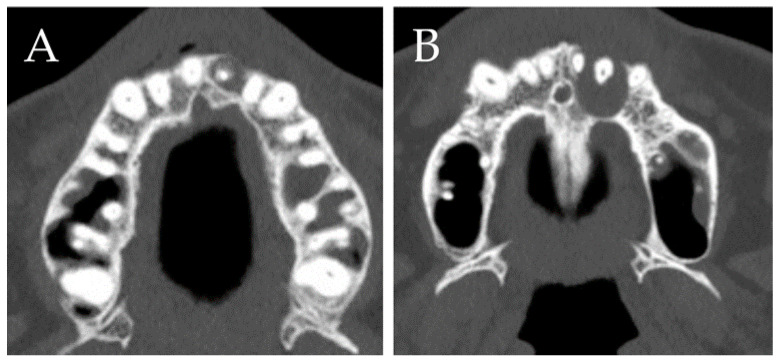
Representative perilesional sclerotic images in axial view of CT: (A) positive perilesional sclerotic sign; (B) negative perilesional sclerotic sign.
